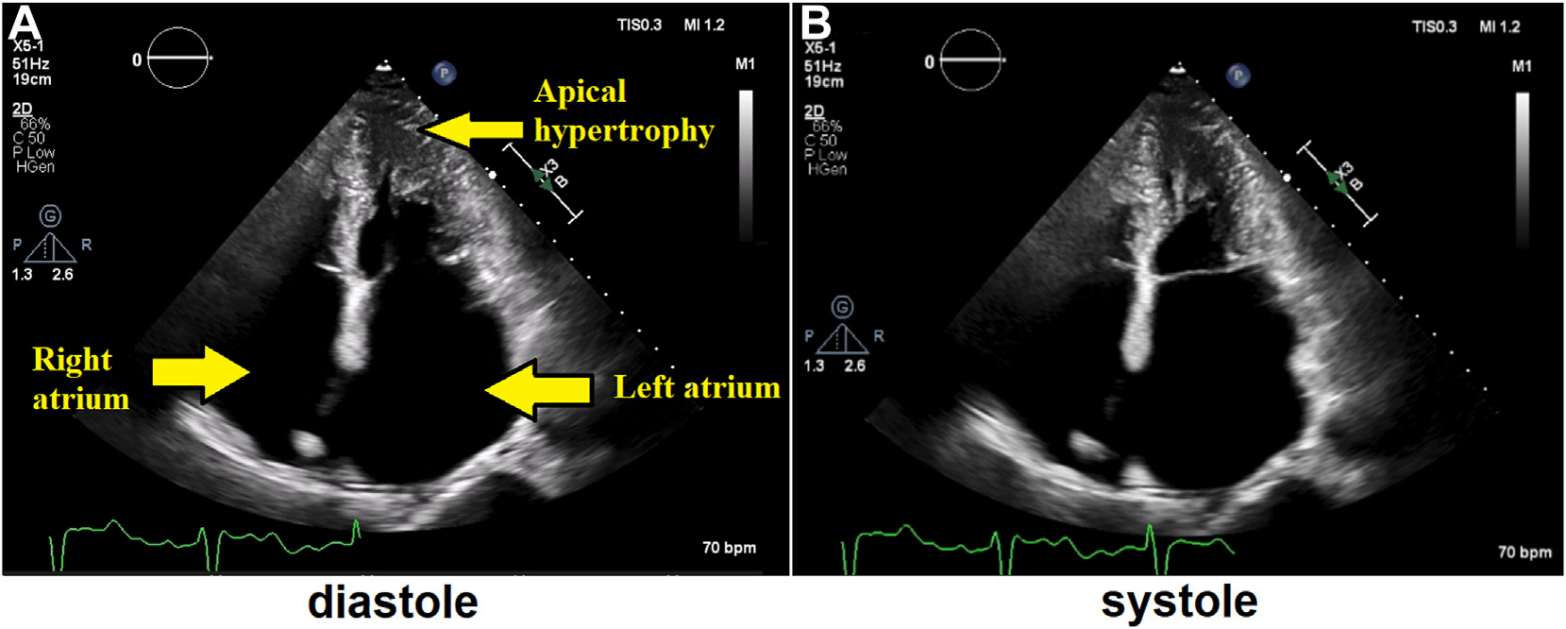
Two-dimensional TTE, apical 4-chamber view in diastole (A) and systole (B), demonstrates progressive mid-to-apical LV wall thickness and severe biatrial dilation.
